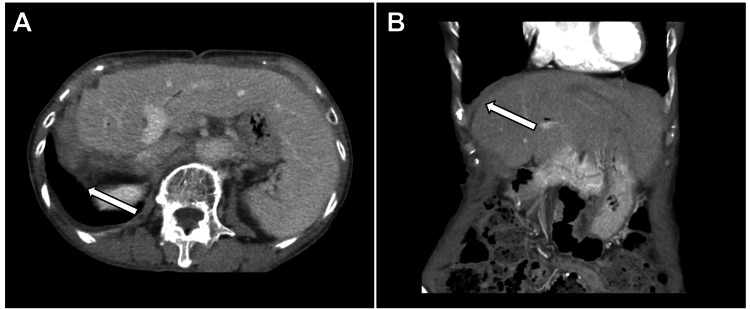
Post-treatment CT images. One month postdischarge contrast-enhanced CT showing the absence of pleural or peritoneal effusion (white arrow) in (A) axial and (B) coronal views.
